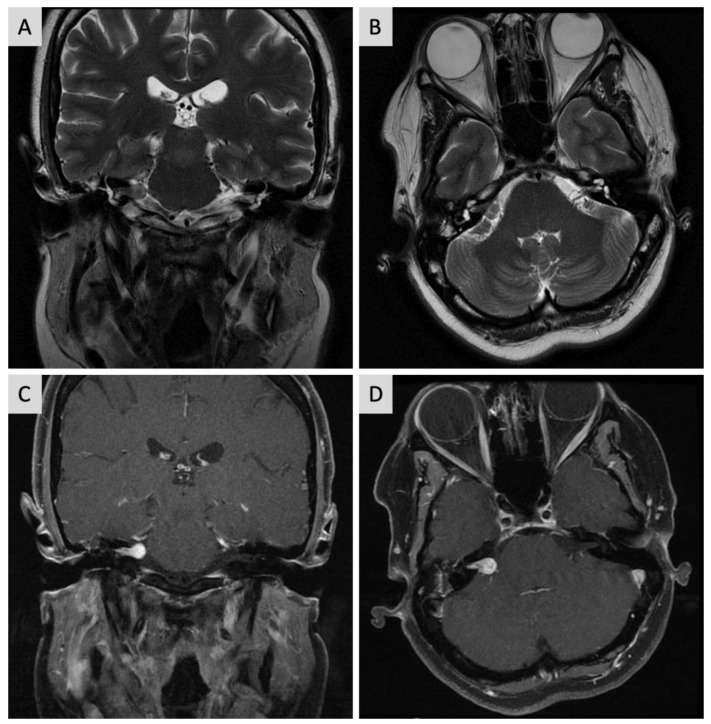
Cranial MRI showing lesion in the right cerebellopontine angle (A) T2-weighted coronal image. (B) T2-weighted axial. (C) T1 contrast-enhanced coronal. (D) T1 contrast-enhanced axial.
